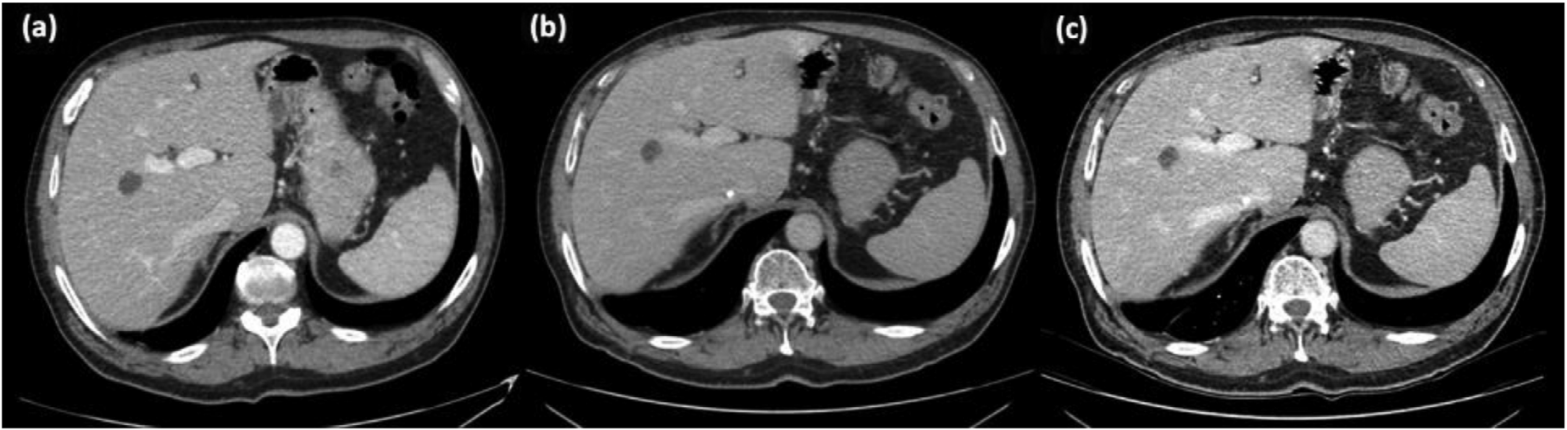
Cross sectional axial slices of the same patient with standard and then reduced ICM contrast dose. (a) Full ICM dose displayed at 70 keV had a CT number measured in the descending aorta of 215.6 ± 17.4 HU. (b) Reduced ICM dose displayed at 70 keV had a CT number measured in the descending aorta of 100.1 ± 18.1 HU. The window width (WW)/window level (WL) was set to 400/40 HU. (c) Reduced ICM dose displayed at 45 keV. The CT number measured in the descending aorta is 218.1 ± 37.3 HU. The WW/WL was set to 536/30 HU.
